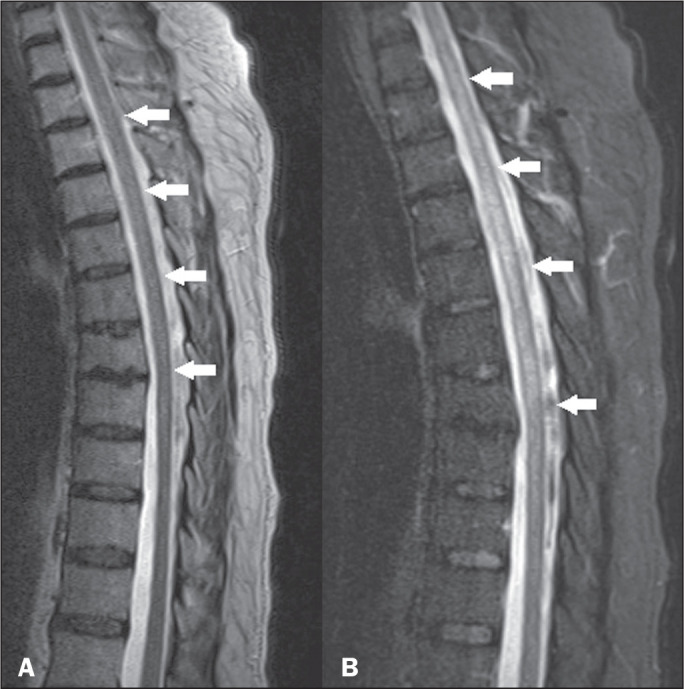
HTLV-1-associated myelopathy. Sagittal T2-weighted and STIR MRI sequences
